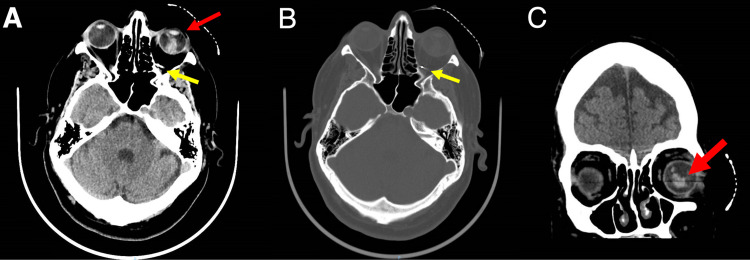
CT of orbits and brain without contrast in the axial (A and B) and coronal (C) planes from the emergency department demonstrating a penetrating open globe trauma (red arrows) along with an orbital foreign body near the orbital apex (yellow arrows).
